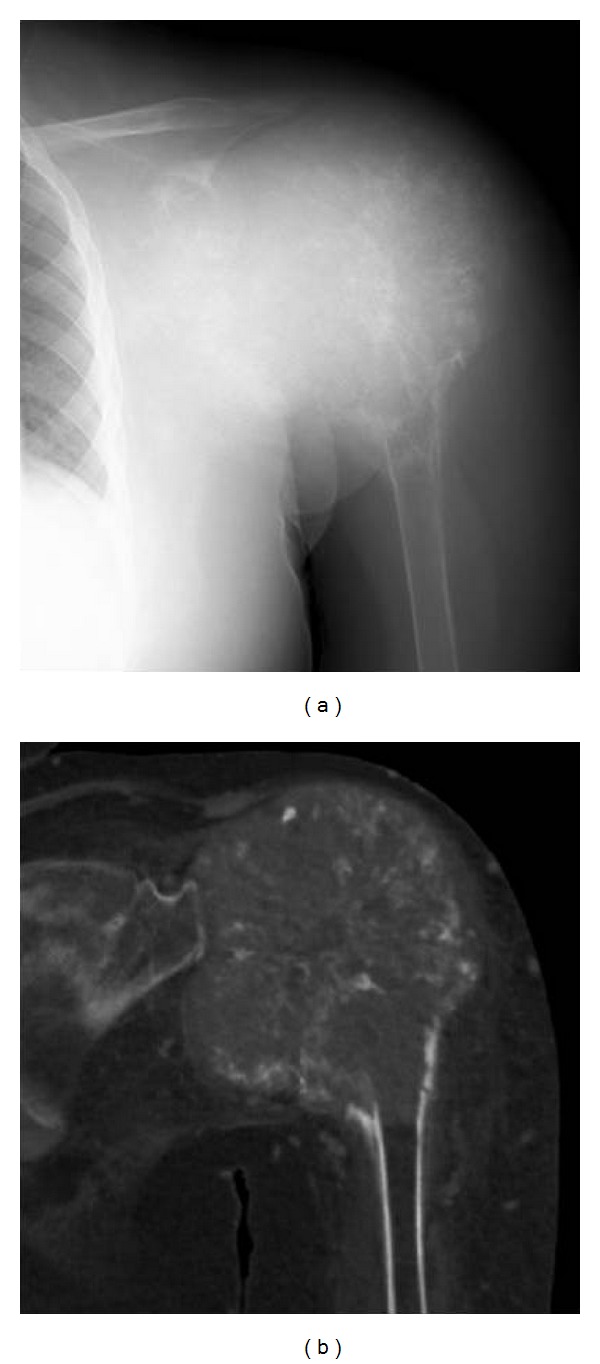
Radiograph (a) and CT reconstruction (b) showed an expanded, destructive lesion of the proximal humerus containing irregular calcifications. There was almost no cortical margin around the lesion.
